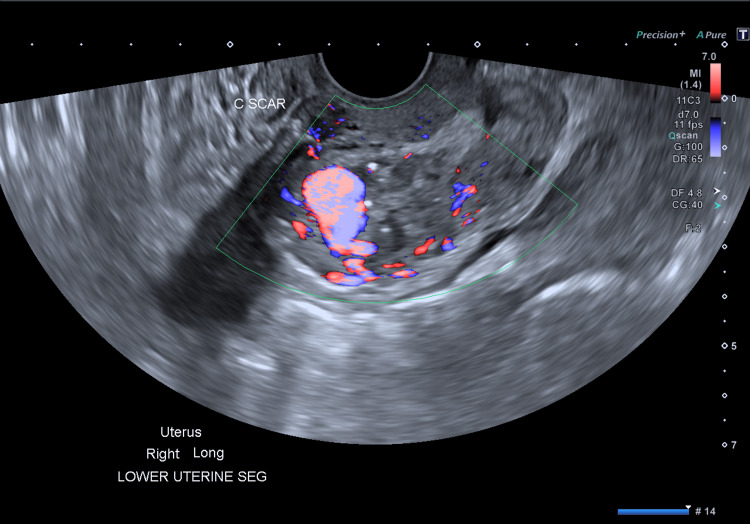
Transvaginal ultrasound of the pelvis demonstrating a 39 × 35 × 29 mm heterogenous mass within the myometrium of the lower uterine segment, arising from the posterior wall. It contains both arterial and venous components with a strong, high-velocity flow.
